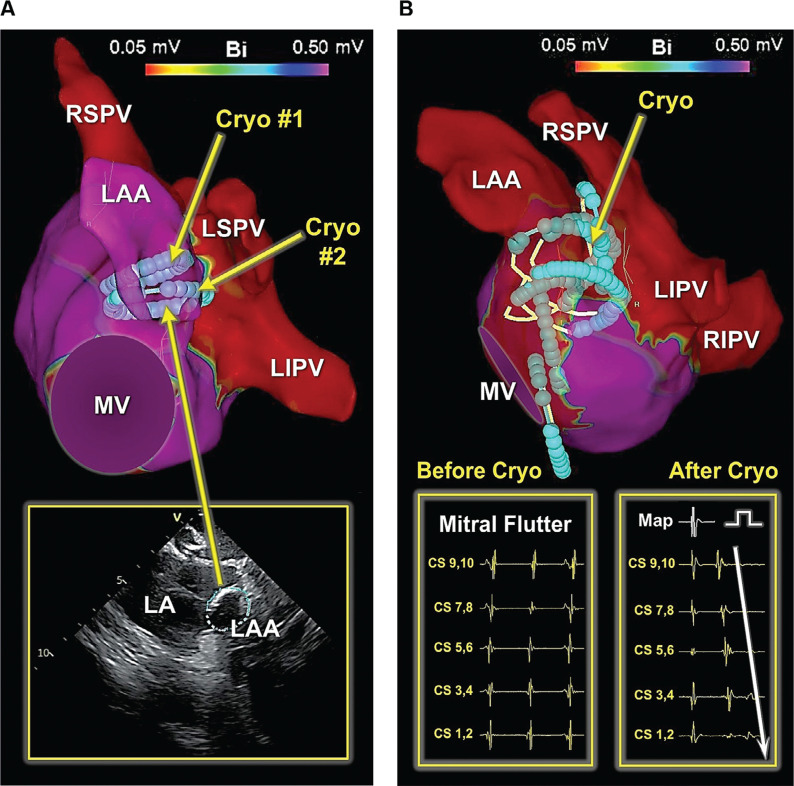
Cryoballoon ablation of the left atrial appendage (LAA) and the mitral isthmus. A: A 3-dimensional (3D) bipolar voltage map of the LA depicting the locations of a 180-second cryoapplication (Cryo #1) followed by a subsequent 120-second bonus application (Cryo #2) to achieve LAA isolation. The position of the second cryoapplication is shown on intracardiac echocardiography (ICE) (inset), which is integrated into the 3D map (CartoSound; Biosense Webster), thereby allowing direct visualization of the balloon at the ostium of the LAA. B: A 3D bipolar voltage map of the left atrium demonstrating the locations of the cryoballoon applications (cryo) for the ablation of the mitral isthmus. Once again, the balloon locations are directly recorded within the 3D map using ICE image integration. The maximum cooling zone of the cryoballoon spans over its entire distal hemisphere, shown in a turquoise color together with the proximal hemisphere appearing in light yellow. Shown here also are the intracardiac recordings during the mitral isthmus flutter (cycle length, 240 ms) prior to cryoablation (left lower inset) and assessment of block across the mitral isthmus post-cryoablation (right lower inset) when pacing anterior to the line of block using a diagnostic mapping catheter. The voltage cutoff is set to 0.05 mV in both maps. Abbreviations: CS, coronary sinus; LA, left atrium; LAA, left atrial appendage; LIPV, left inferior pulmonary vein; LSPV, left superior pulmonary vein; MV, mitral valve; RIPV, right inferior pulmonary vein; RSPV, right superior pulmonary vein.
